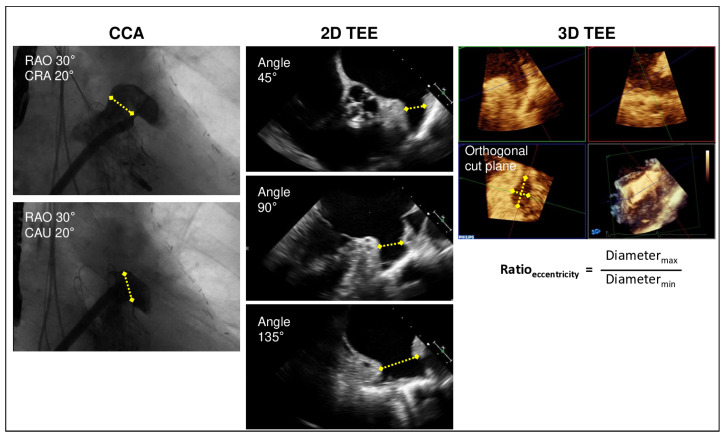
Sizing of LAA landing zone. Measurements of diameters (yellow arrows) by conventional cardiac angiography (CCA) in two projections: by 2D transesophageal echocardiography (TEE) in 45°, 90°, and 135° views as well as 3D TEE cut-plane view of the LAA landing zone and calculation of 3D eccentricity index as the ratio of the maximal and minimal diameters.
